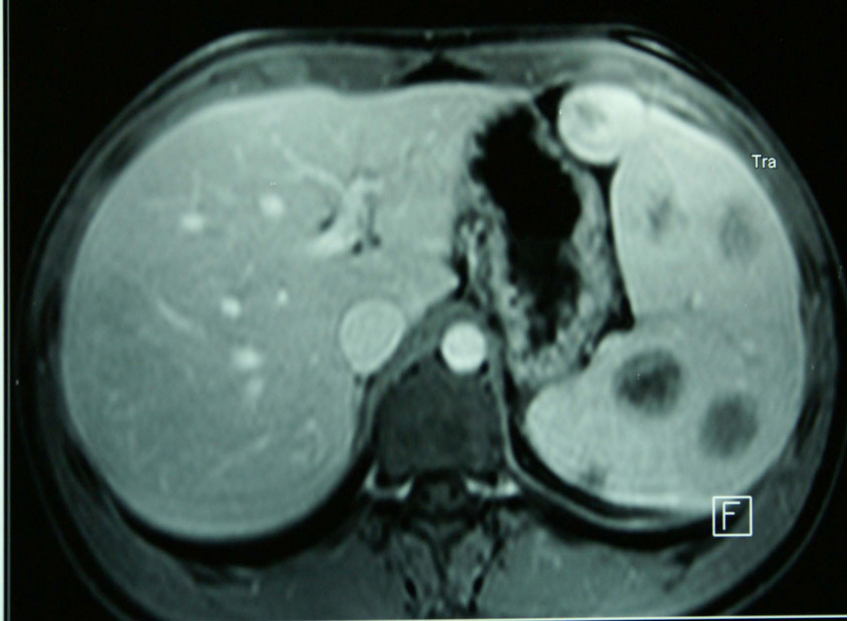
Magnetic resonance imaging (MRI) showing the splenomegaly and the hemangioms.
